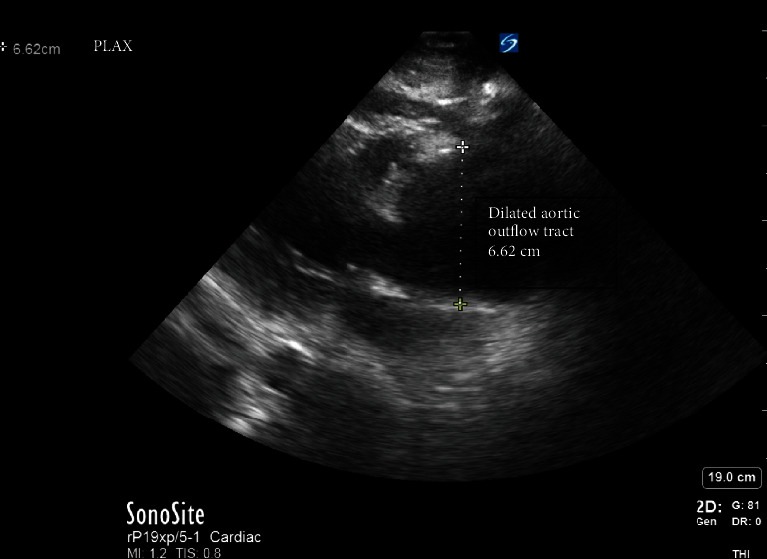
Parasternal long axis view of the heart, demonstrating a dilated aortic outflow tract measuring 6.62 cm during diastole. Normal size of the aortic outflow tract is less than 4 cm.
